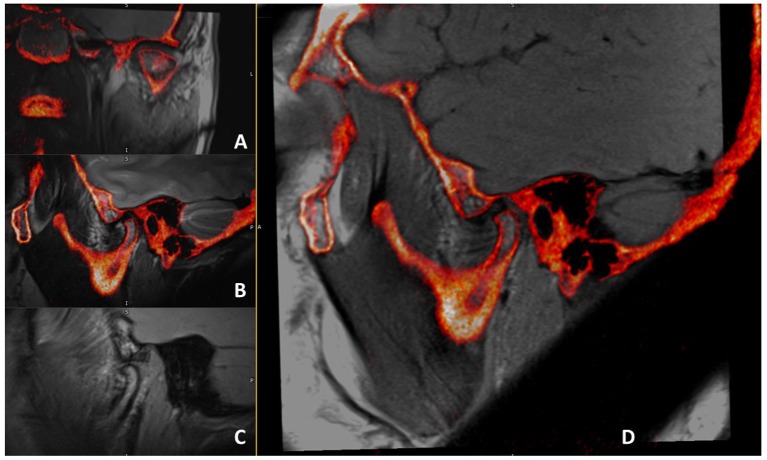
Multiple MRI sequences and CBCT images after registration. A: Oblique coronal PD-weighted MRI-CBCT registered image; B: Oblique sagittal T2-weighted MRI-CBCT registered image; C: Open mouth oblique sagittal PD-weighted MRI only. D: Oblique sagittal PD-weighted MRI-CBCT registered image.
